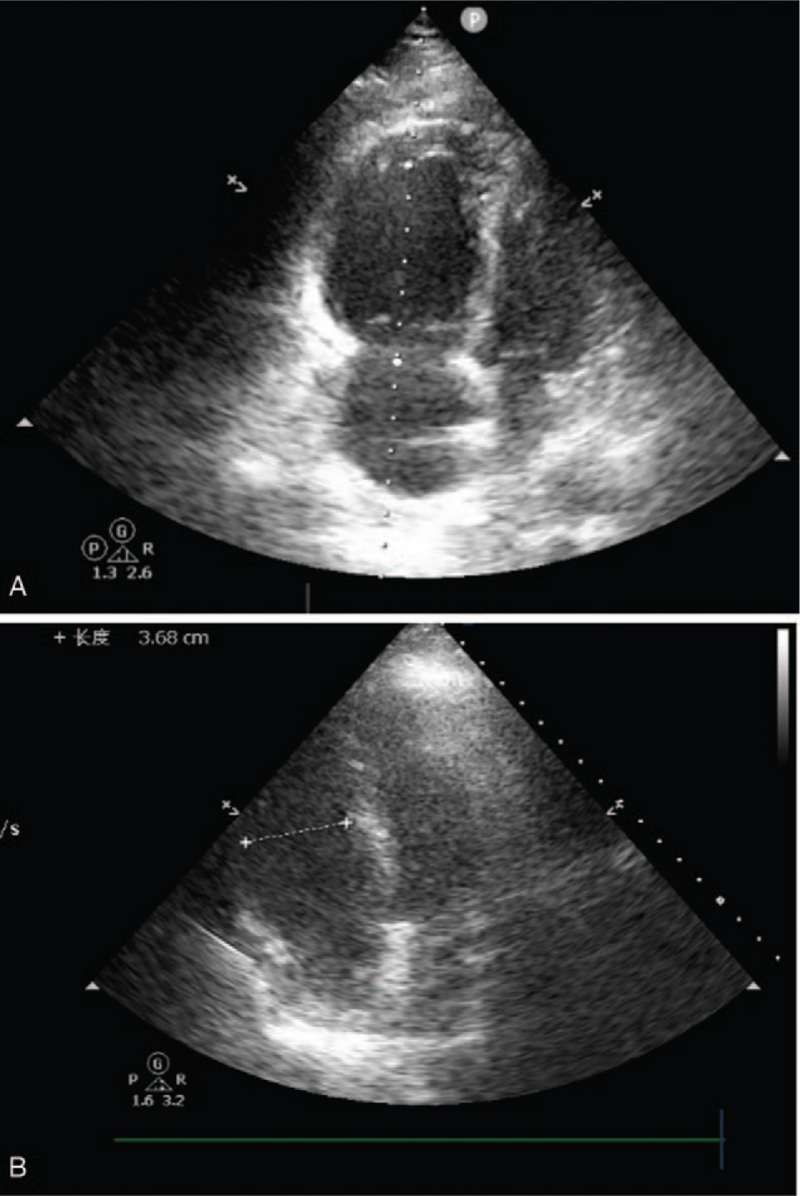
Bedside cardiac ultrasound of the patient before and after carrying out ECMO. (A) Bedside cardiac ultrasound showed right heart expansion (RV 44 mm) before carrying out ECMO. (B) Bedside cardiac ultrasound showed right heart size dropped to normal (RV 36 mm) after carrying out ECMO. ECMO = extracorporeal membrane oxygenation.
